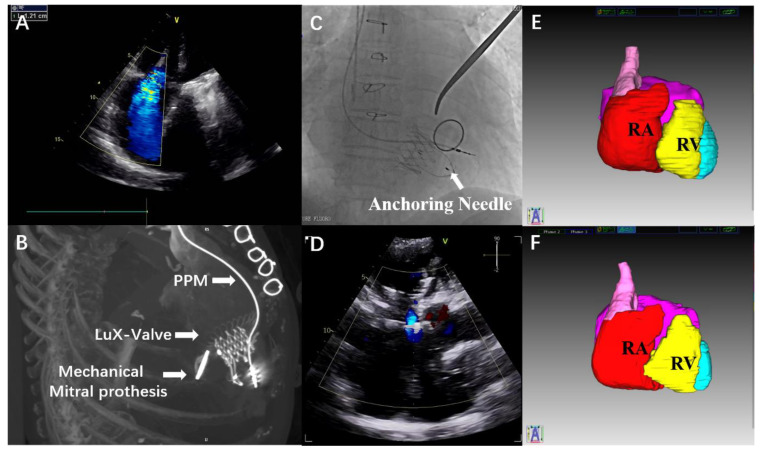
Procedural Steps of LuX-Valve Transatrial Tricuspid Valve Replacement. (A) Pre-operative transthoracic echocardiogram discloses severe tricuspid regurgitation. (B) Post-operative computed Tomography (CT) reconstruction shows the implants of the patient. (C) The fluoroscopy after releasing the anchoring needle. (D) A transthoracic echocardiogram shows trivial paravalvular leakage at 4-year follow-up. (E) Three-dimensional CT reconstruction of the whole heart with volumetric assessment of RV (shaded yellow). (F) Three-dimensional CT reconstruction indicating a slight increase in RV at 4-year follow-up. PPM = permanent pacemaker; RV = right ventricle.
